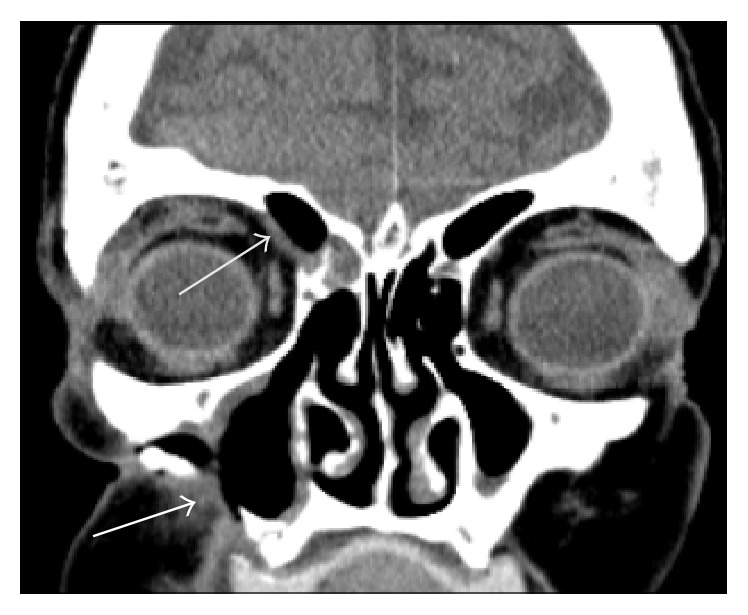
Coronal section CT image showing bony destruction in the lateral wall of the maxillary antrum with bone appearing to show some erosion and thickening. Bony dehiscence seen on the right superior orbital plate (white arrows) (courtesy of Dr. Tauseef Ashraf, Department of Radiology, Pilgrim Hospital, United Lincolnshire Hospitals NHS Trust).
